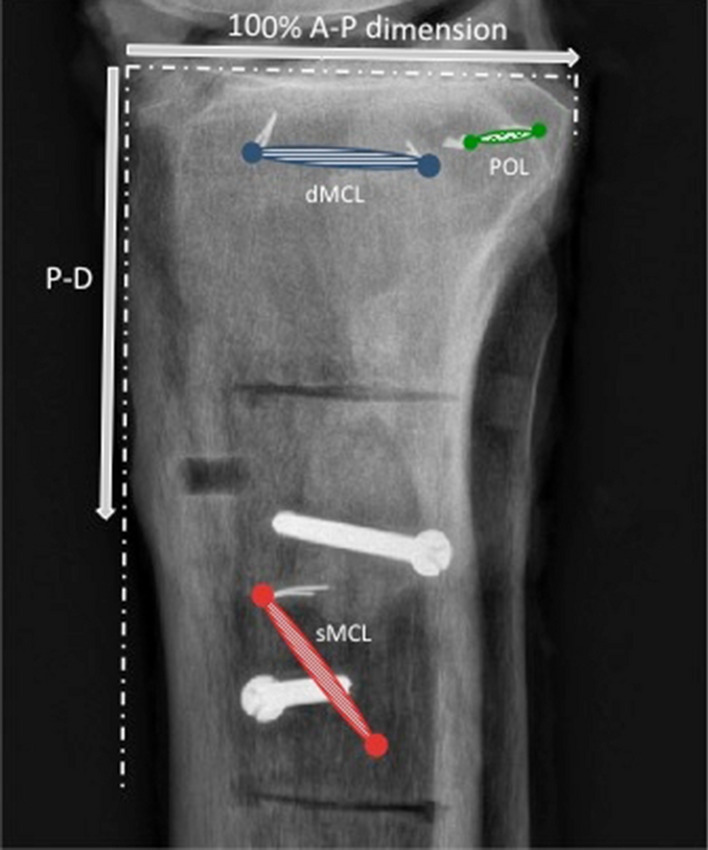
Tibial attachments of the superficial MCL (sMCL), deep MCL (dMCL) and the posterior oblique ligament (POL) as seen radiographically, in relation to the normalisation as a percentage of the A-P size (100%) of the medial tibial plateau, in both A-P and P-D directions. The arrow related to P-D measurement uses the same 100% length as the A-P measurements. Saw cuts for the elevation of a block of bone with the distal attachment of sMCL can be seen. The 2 distal screws have been used to fix the bone block back in place
